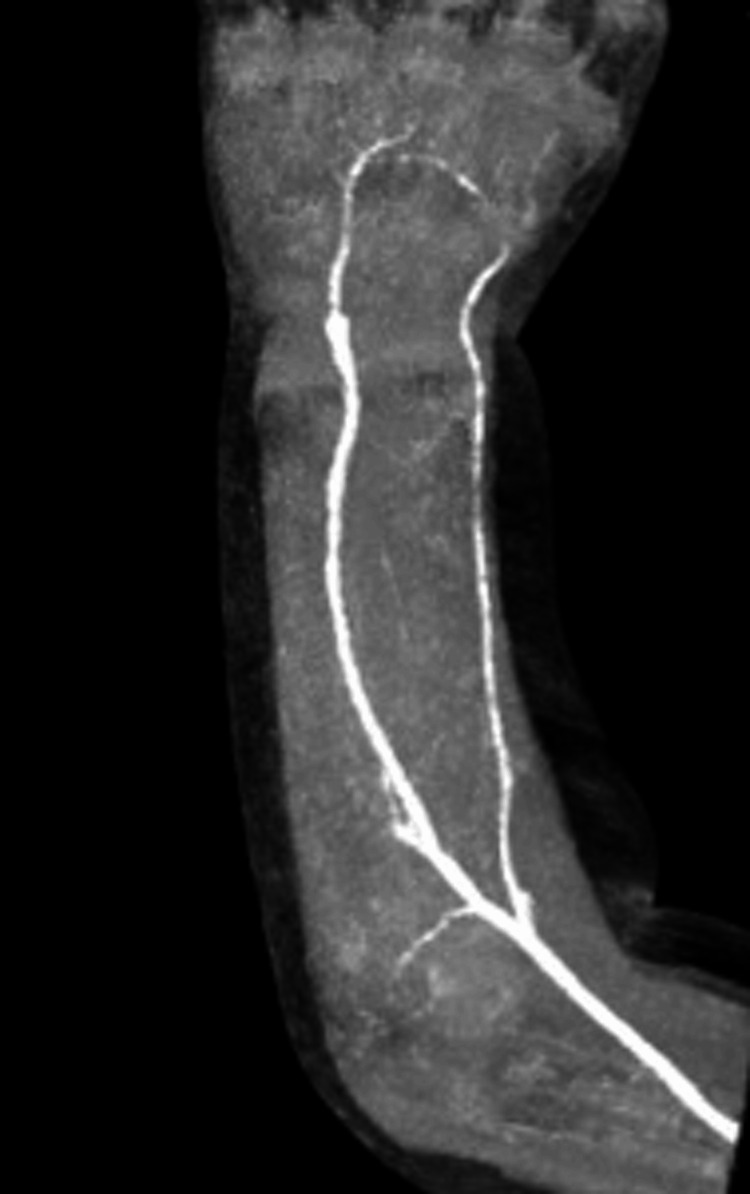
Contrast-enhanced CT of the right forearm four months after surgery. The reconstructed ulnar artery is patent, and there is no impairment of blood flow to the hand.
